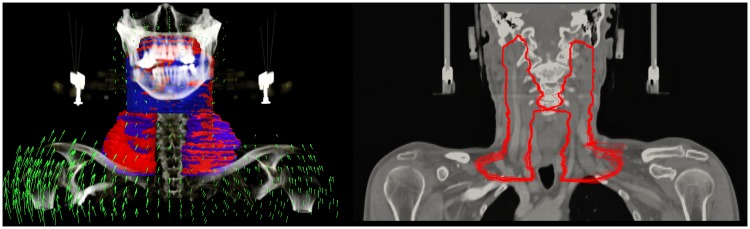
The Calculation of displacement vector fields (DVFs) allows a propagation of the CTV contours from planning image to daily control images. Left: The arrows (green) represent an exemplary DVF of the transformation between a planning CT image and a control CT image. The CTV contour (blue) was propagated to fCTV of the fraction (red). Right: the fCTV of patient #19 propagated to all control CT images are displayed on the planning CT image.
